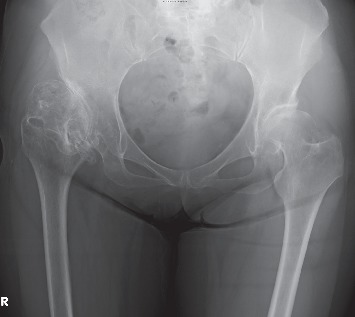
Anteroposterior radiograph of the both hip joints.
